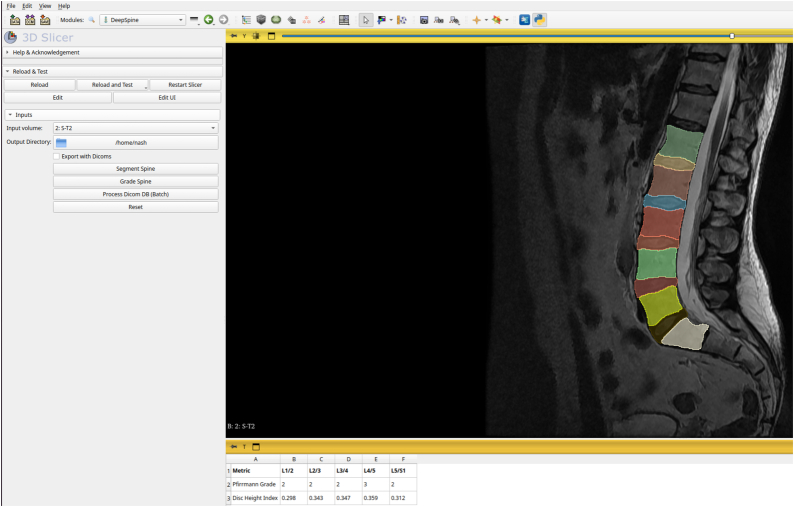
3D Slicer extension interface, showcasing the segmentation, grading, and batch processing modules. The extension enables automated analysis of lumbar spine MRI, including vertebral body and disc segmentation, grading, and Disc Height Index calculation, streamlining large-scale data processing for research and clinical applications.
